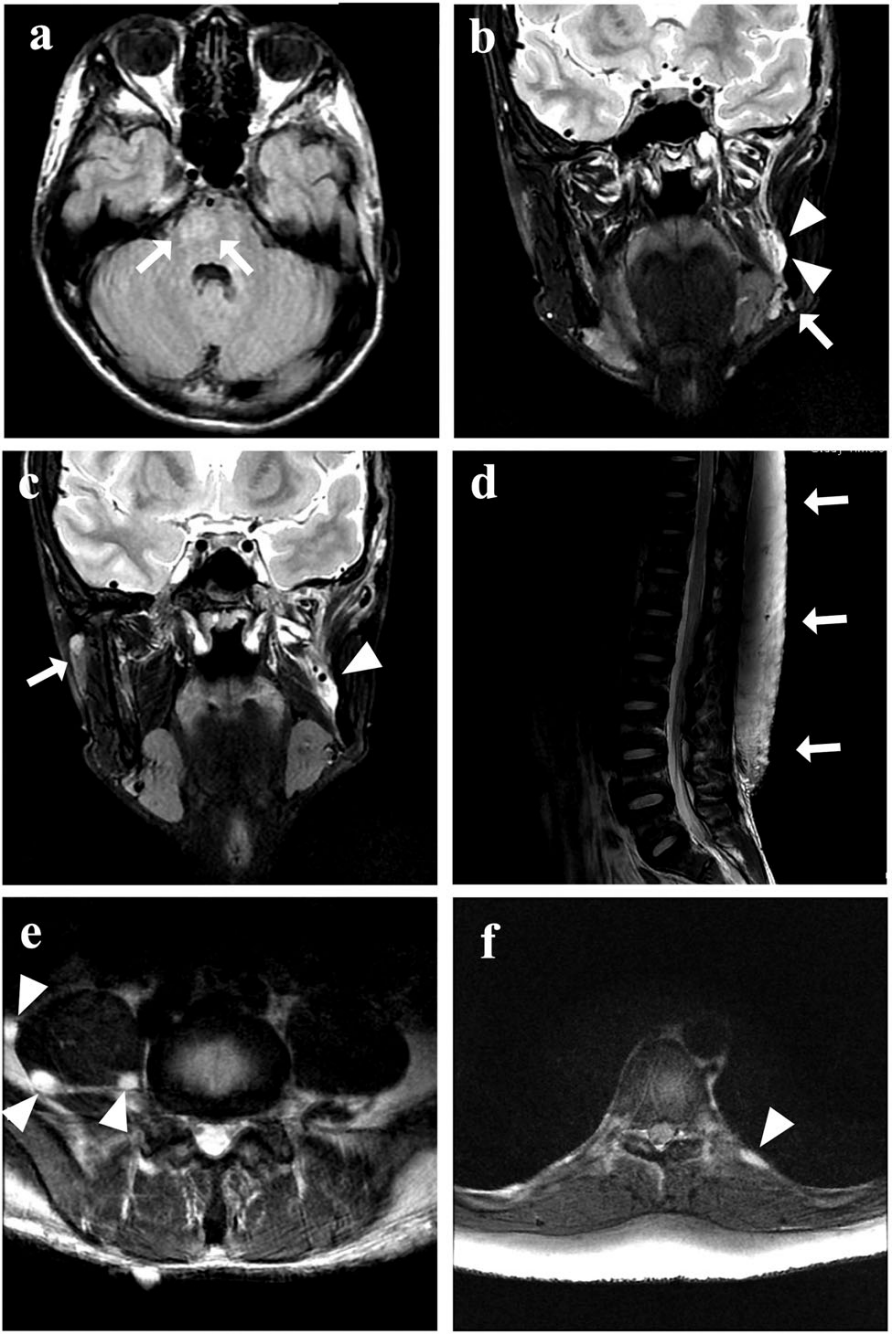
MRI findings. a Brain MRI axial FLAIR image at age 16. Arrows indicate hamartomatous lesions. b, c Coronal T2-weighted brain MR image at age 16. The arrows indicate nodules suspected to be neurofibromas in the left mandibular (b) and right buccal (c) subcutaneous areas. Arrowheads indicate neurofibromas. d Sagittal T2-weighted view of a lumbar MR image at age 22. The arrows indicate lumbar subcutaneous adenomas. e, f Axial T2-weighted views of a lumbar MR image at age 22. The arrows indicate neurofibromas around the bilateral psoas major muscle (e) and along the pleura (f).
